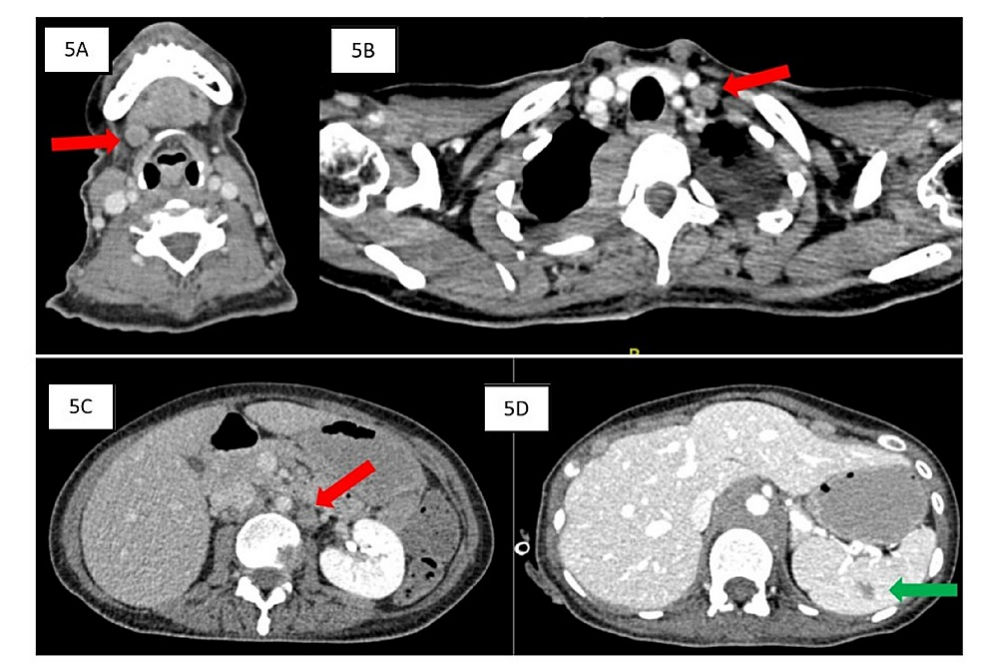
Contrast-enhanced CT images in axial sections. Multiple enlarged lymph nodes (red arrows) are seen at right submandibular (A), left supraclavicular (B), and para-aortic (C) regions. An ill-defined hypodense lesion at the spleen (D, green arrow) could represent lymphomatous infiltration.
